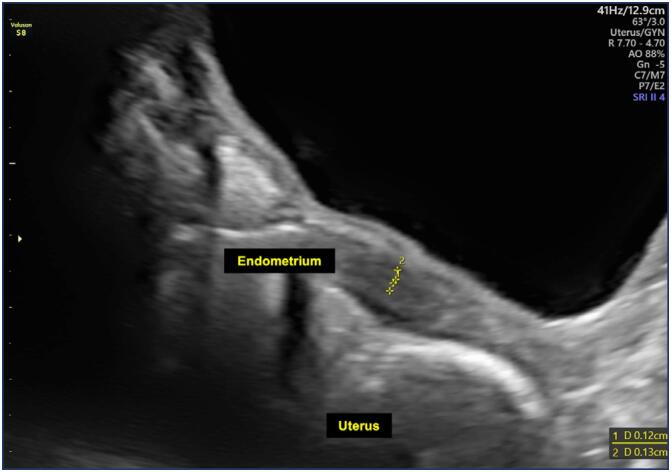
Pelvic ultrasound image showing a uterus with prepubertal dimensions and an endometrial thickness of 1.2 mm, indicated by the dashed line (Ultrasound device - Voluson S8 – GE Health Care)
